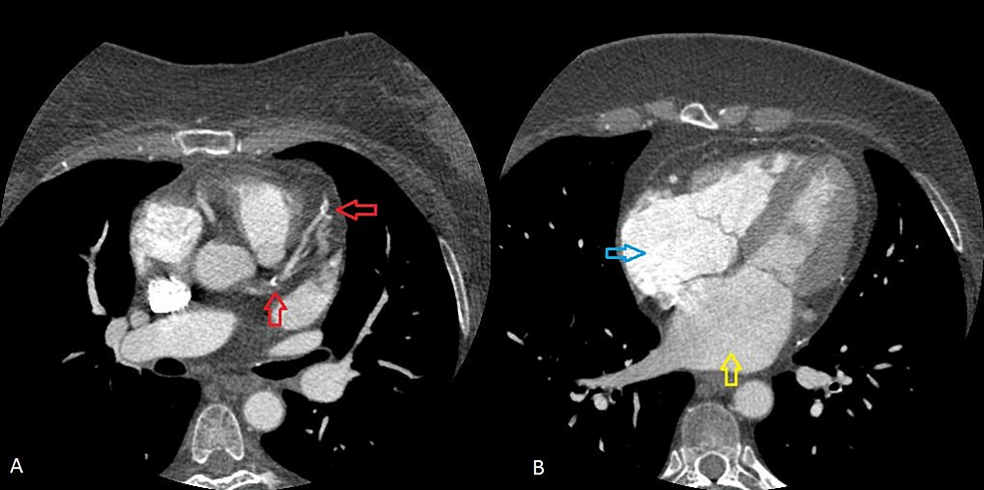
Cardiac CT scan. A: red arrows indicate coronary artery calcifications.  B: right and left atrial enlargement is seen (blue and yellow arrows, respectively).
